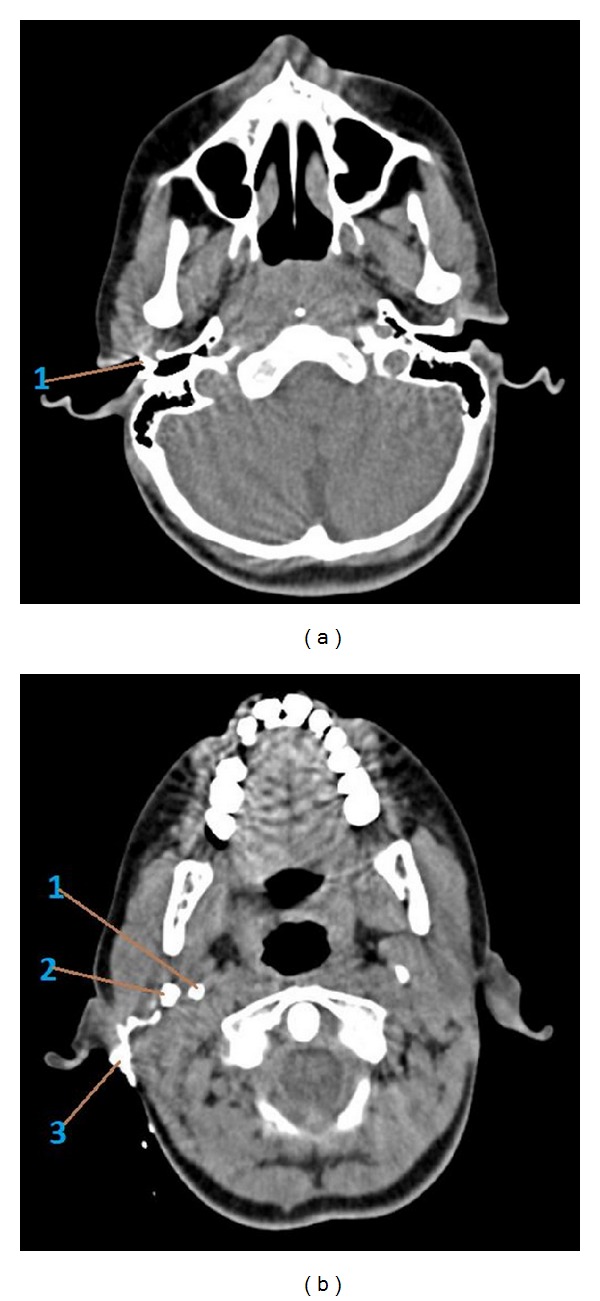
(a) CT fistulogram axial section showing the opening into the external auditory canal at bony cartilaginous junction (marked as 1). (b) CT fistulogram axial section (lower section to Figure 4(a)) showing the bifurcation of the fistula tract. (1) Styloid process, (2) main fistula tract going towards external auditory canal, and (3) bifurcation going to the postaural region with spillage of contrast.
